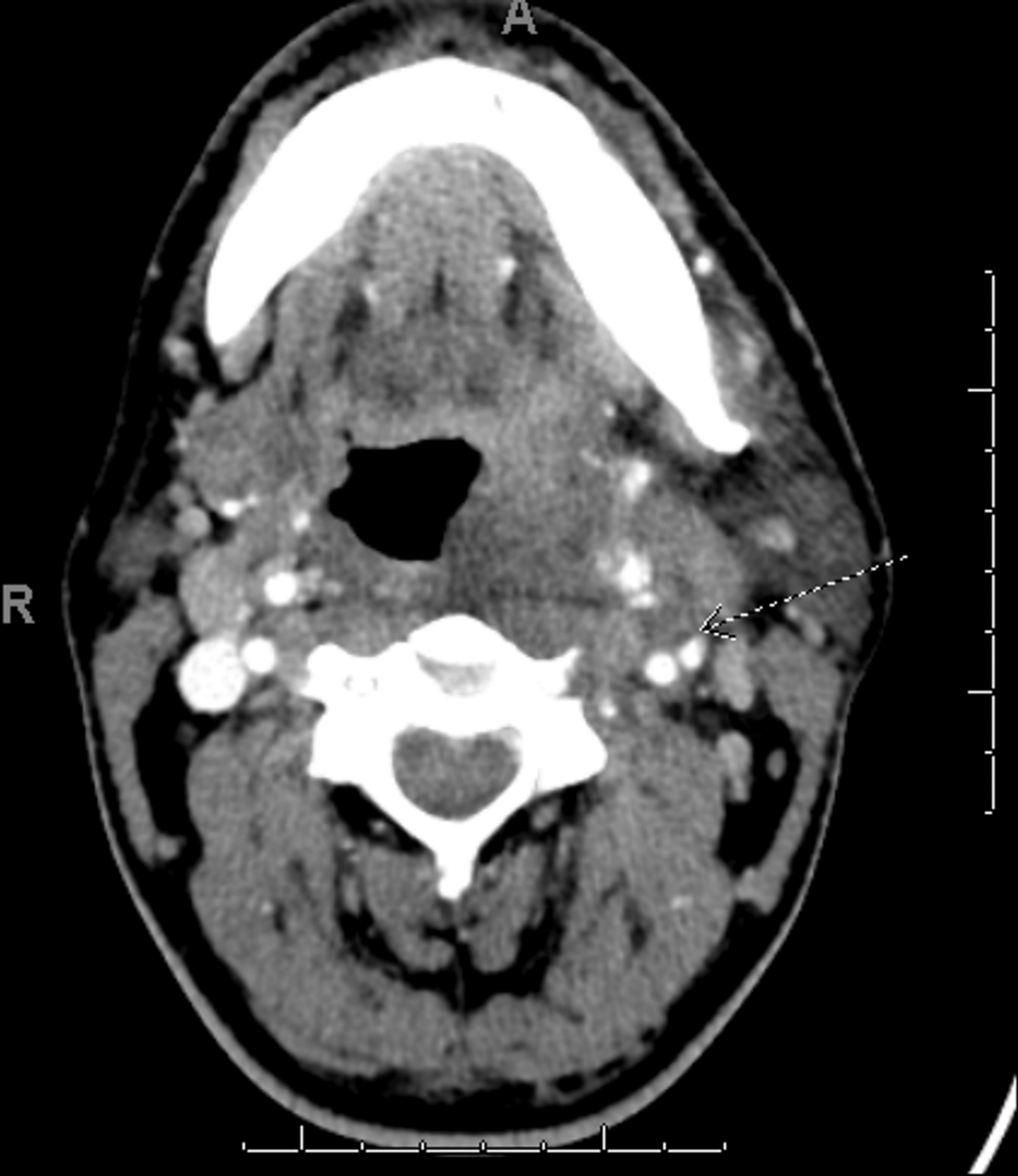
Initial contrast-enhanced axial CT scan showing internal jugular vein (IJV) filing defect indicating a partial occlusion of the left IJV as indicated by the arrow.
